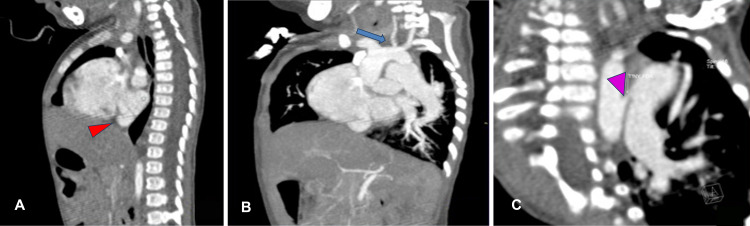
Contrast cardiac CT scan (sagittal and coronal view) of the 11-month-old boy (A) Sagittal reconstruction showing membrane of cor triatriatum (red arrowhead). (B) Oblique coronal reconstruction showing hypoplastic left common carotid artery (blue arrow). (C) Oblique coronal reconstruction showing tiny PDA. PDA: patent ductus arteriosus
